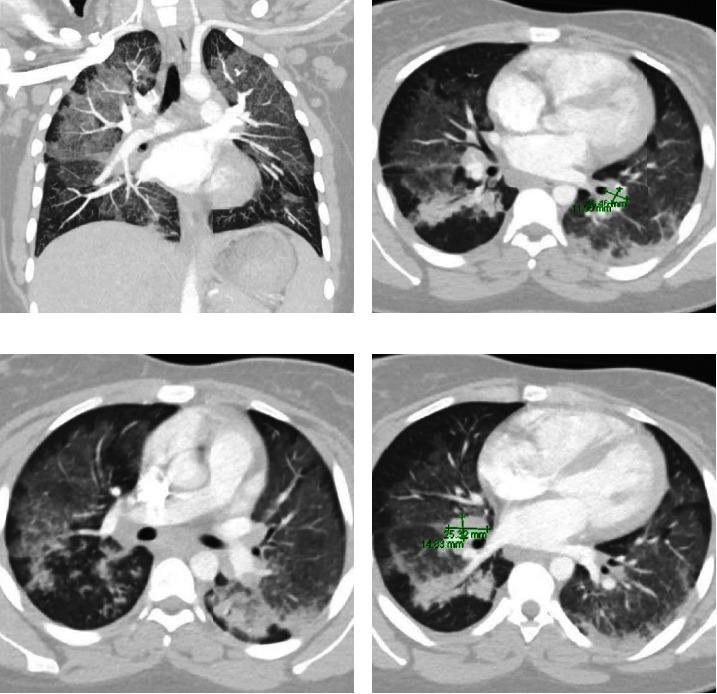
Computed topography (CT) scan showing diffuse ground glass opacities (a–d). CT scan also shows mediastinal lymph node enlargement (b, d).
